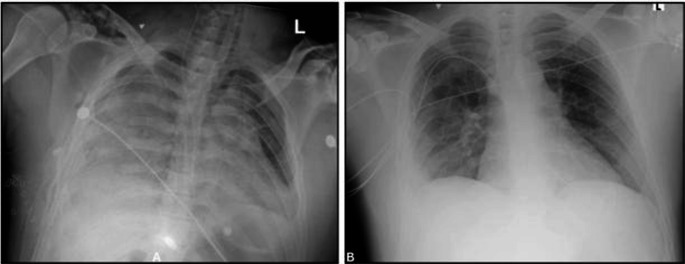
Chest X-rays of a patient with a gunshot injury to the right hemithorax. A – Postoperative Day (POD) 0: Extensive pulmonary contusion, multiple rib fractures, subcutaneous emphysema extending into the neck, and visible retained ballistic fragments. A projectile is seen projected over the thoracic spine, located subcutaneously in the posterior thorax. B – POD 73: Following surgical extraction of retained bullets from the mandible and subcutaneous tissues of the back, near-complete radiographic resolution of the pulmonary injury is demonstrated
